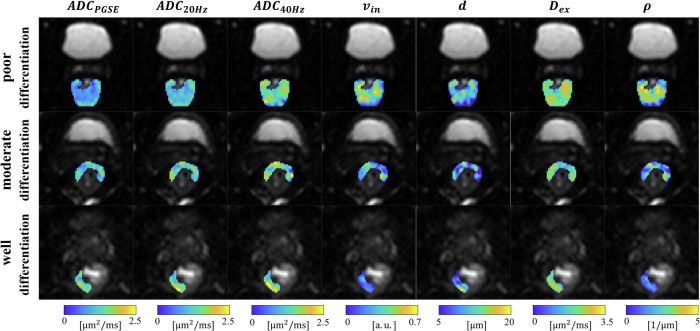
The imaging findings of three representative rectal tumors, with poor, moderate, and well differentiation grades, respectively. The background of all subplots is the PGSE image with b = 1000 s/mm^2^. The pseudo-color image displays the color-coded distribution of parameter values for a specific imaging metric, including three time-dependent ADCs, $${v}_{{in}}$$, $$d$$, $${D}_{{ex}}$$, and $$\rho$$, within the tumor lesion area (i.e., ROI). The plane of DWI is axial, and the hyperintense structure, especially visible on the maps with poor or moderate differentiation, is bladder. ADC, apparent diffusion coefficient; $${v}_{{in}}$$, intracellular volume fraction; $$d$$, cell diameter; $${D}_{{ex}}$$, extracellular diffusivity; $$\rho$$, image-derived cellularity; DWI, diffusion-weighted image; PGSE, pulsed gradient spin-echo
